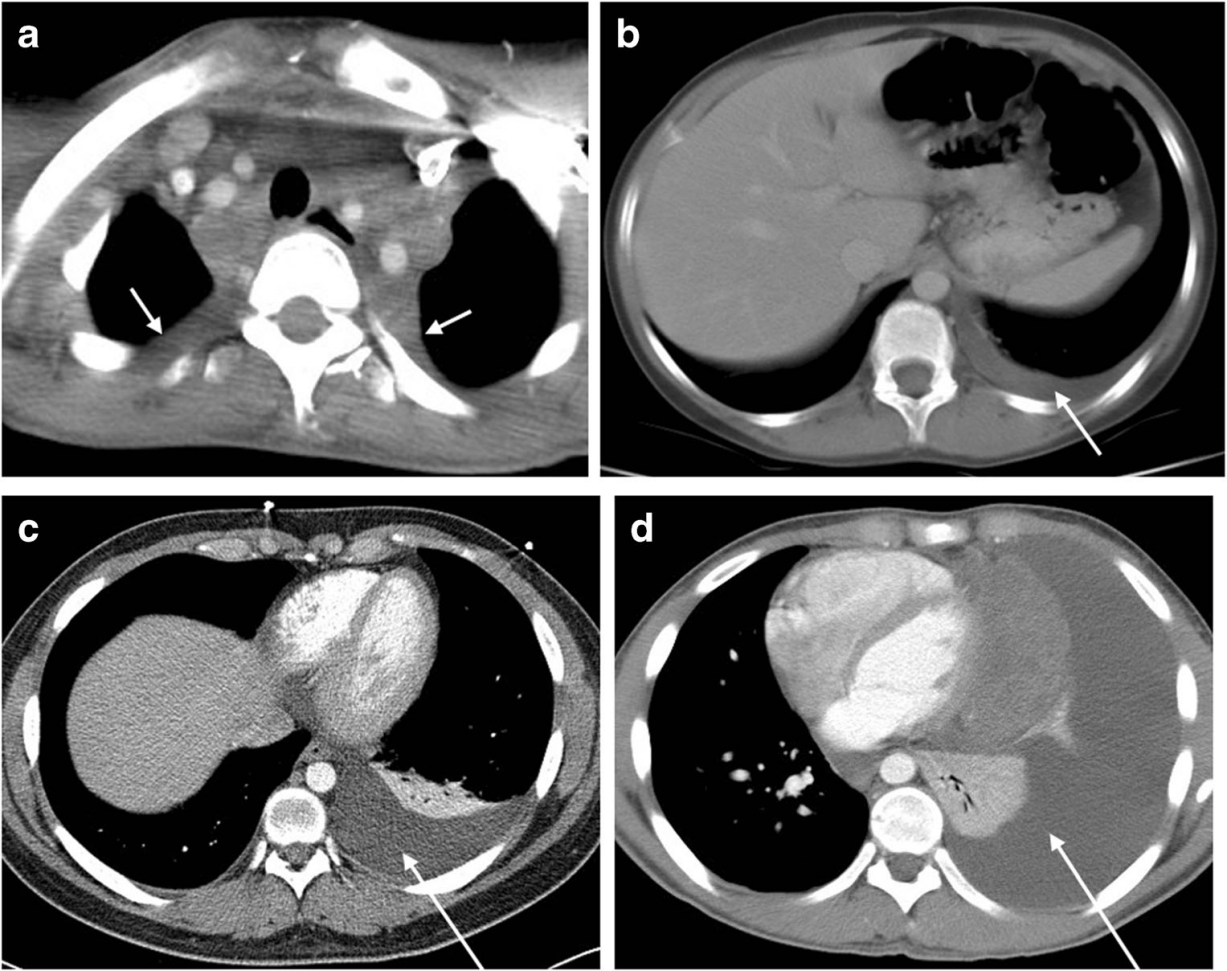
Effusion categories. a Trace effusion (*arrows*), with at least 1 cm of fluid inferiorly or 1 cm at the apices, as seen here on axial CT image in a 15-year-old girl. The majority of trace effusions were at the apex (129 of 217). This is a distinct finding associated with large mediastinal masses and might reflect transudation from small focal lymphatic channels. b Small effusion (*arrow*), seen here in an 11-year-old girl on axial CT image, is any effusion 1–3 cm in size. c Moderate effusion (*arrow*) is any effusion >3 cm in size that reached to mid-thoracic level, as seen in a 16-year-old girl on axial CT image. d Large effusion (*arrow*), any effusion that extends from the lung base to the apex and displaces heart and mediastinum toward the opposite side, is seen here on axial CT image in a 14-year-old boy
